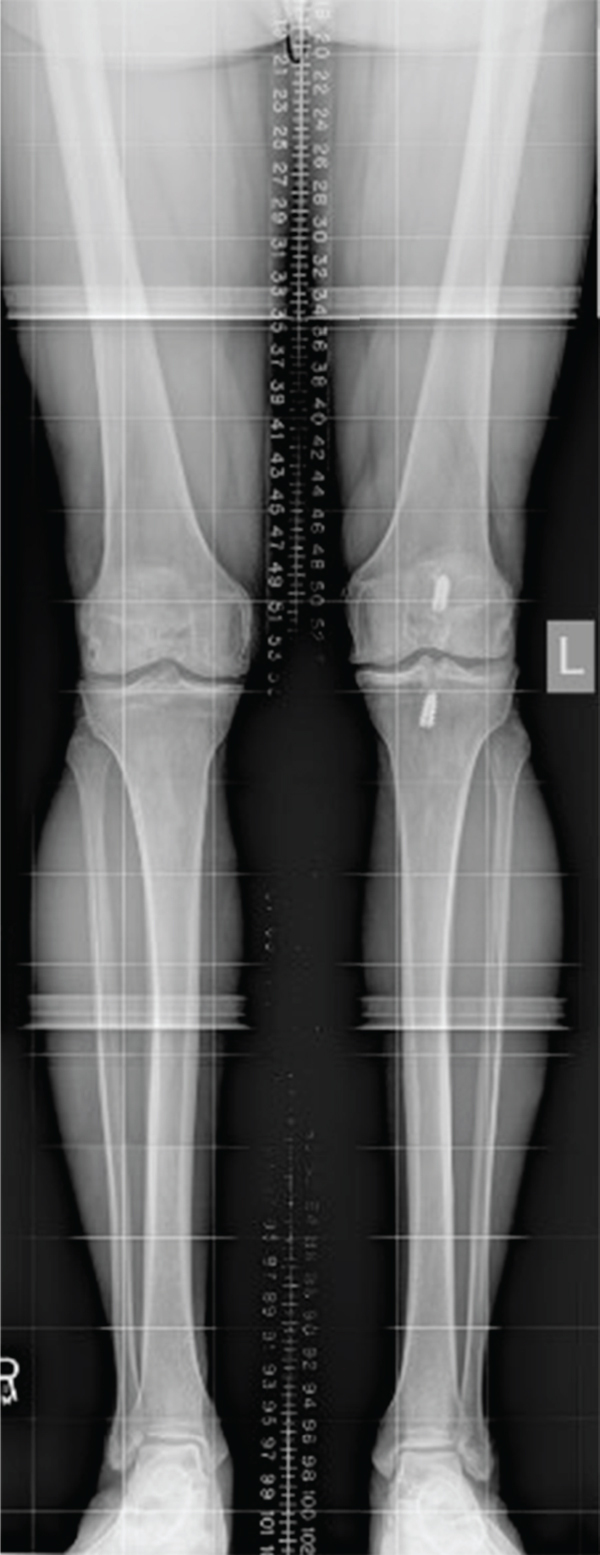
Hip to ankle long-leg standing radiograph demonstrating bilateral symmetric 3° genu valgum.
